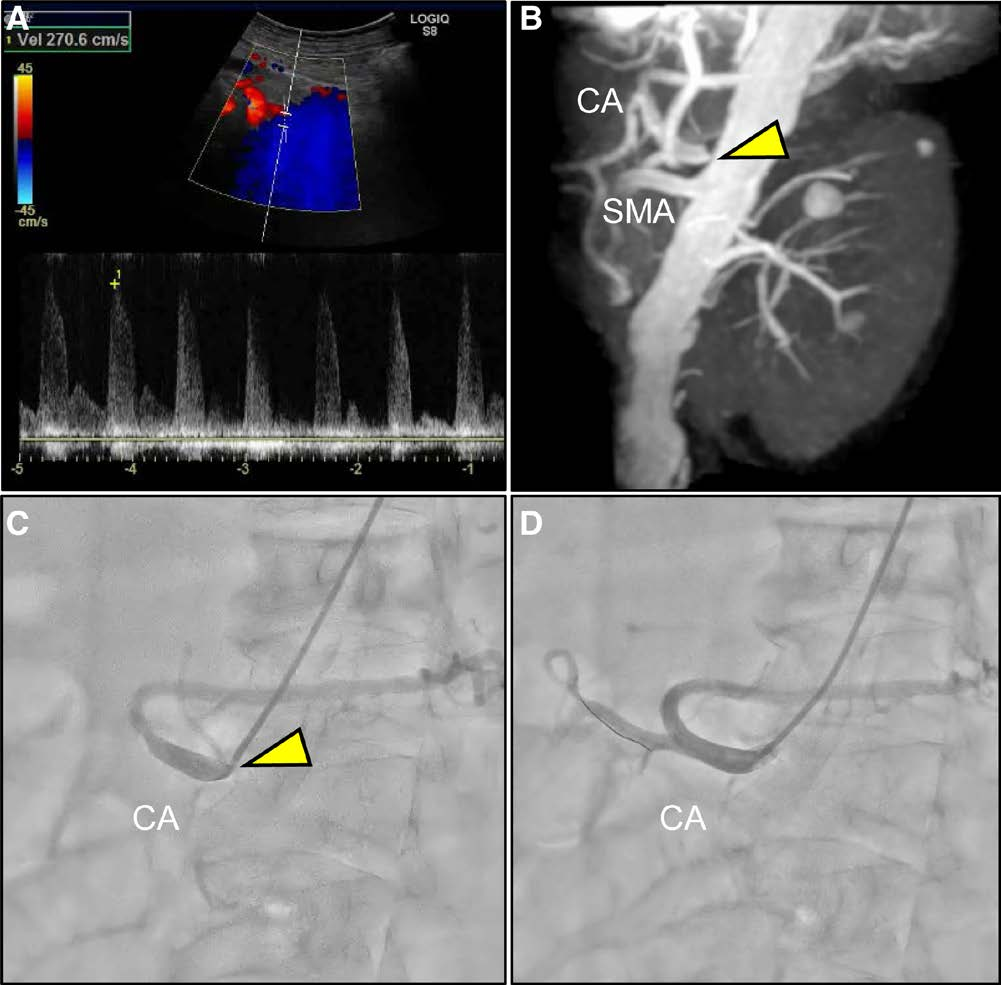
Multimodality imaging of stenosis of the CA. (A) Duplex ultrasonography of the CA reveals peak systolic velocity of 270 cm/s. (B) Noncontrast-enhanced magnetic resonance angiography in sagittal view reveals high-grade stenosis of the celiac trunk origins at the aorta (arrowhead). Selective angiography of the CA in left anterior oblique view (C) before and (D) after endovascular treatment. Note the subtotal stenosis at the origin of the celiac trunk (arrowhead). CA = celiac artery, SMA = superior mesenteric artery.
